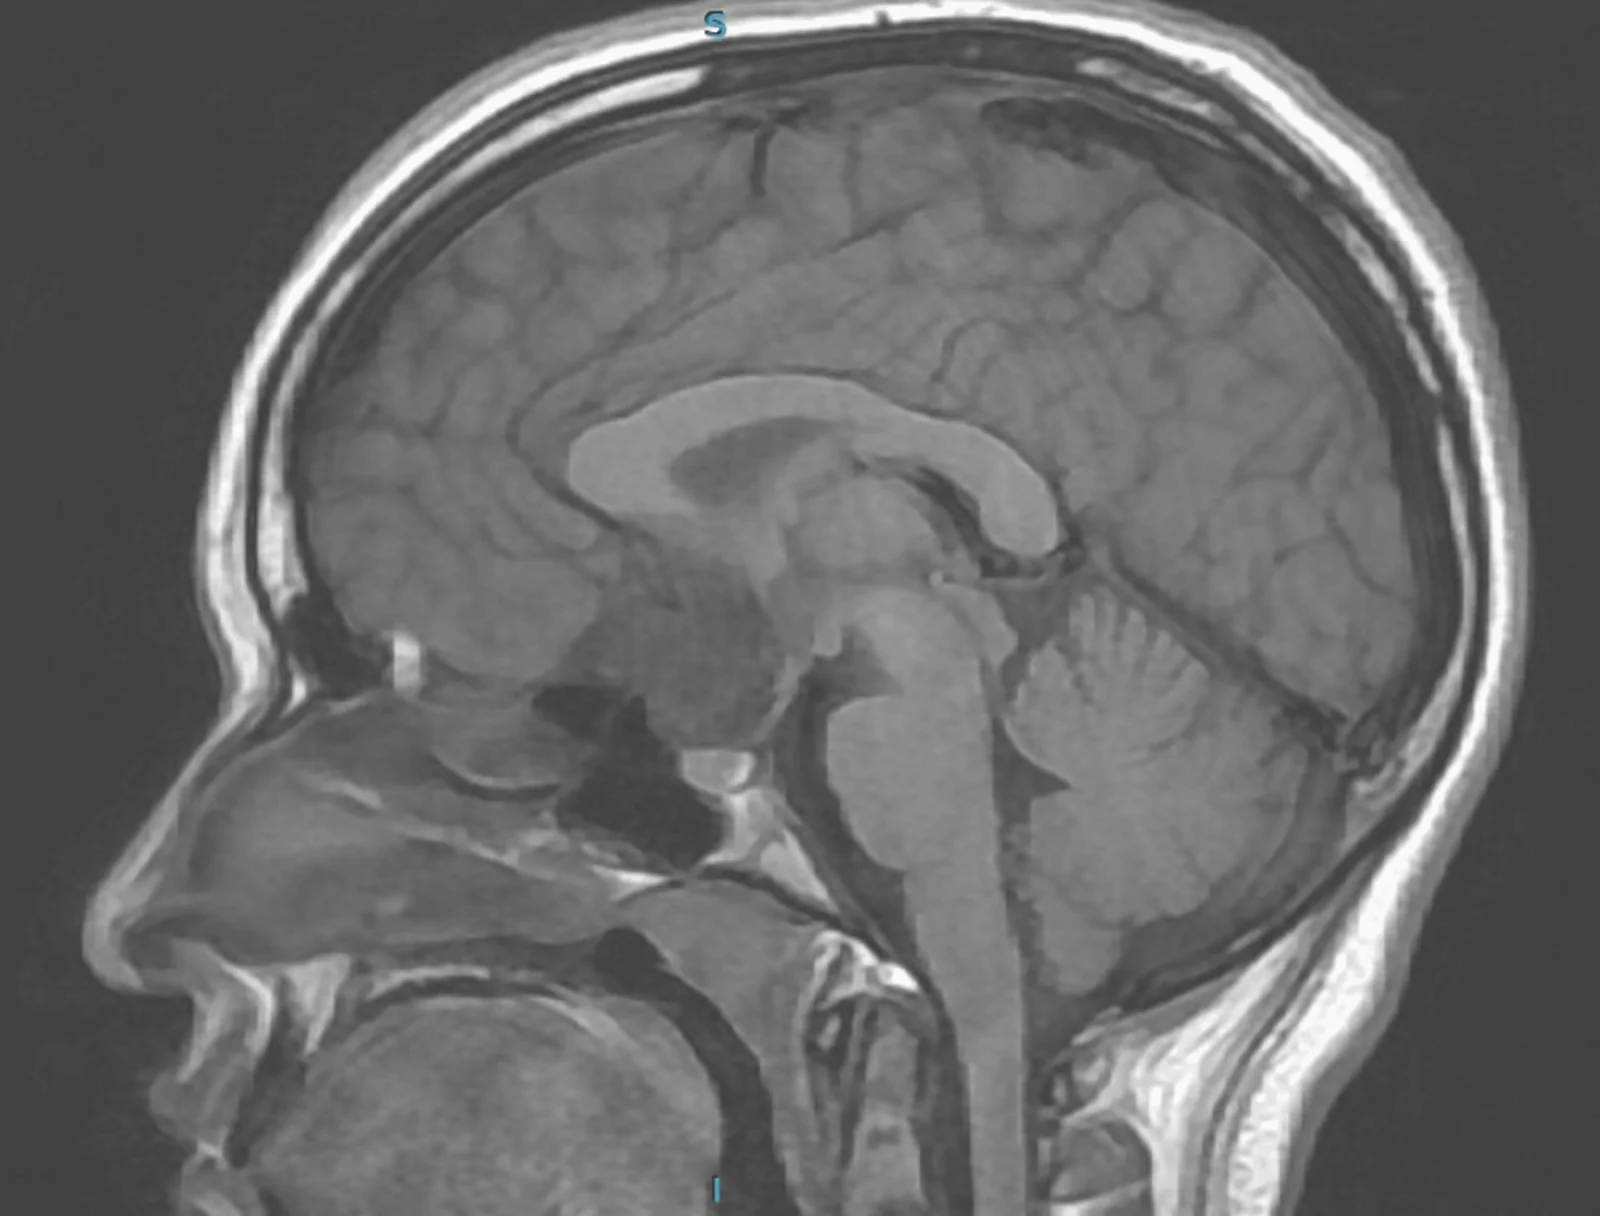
Sagittal T1-weighted MRI of the sellar and suprasellar region A well-defined, hypointense cystic lesion causes superior displacement of the optic chiasm and compression of the infundibulum. MRI: magnetic resonance imaging
